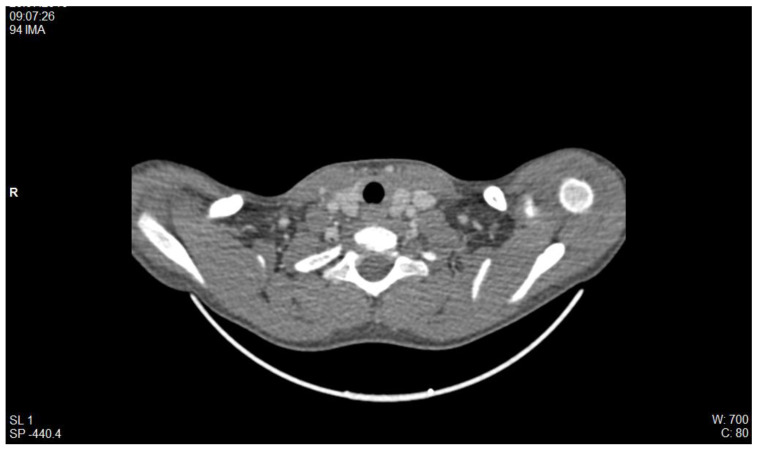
CT scan, evaluation 2 months after the acute episode reveals persistence of thrombosis in the right IJV without progression (personal collection Nephrology Department).
